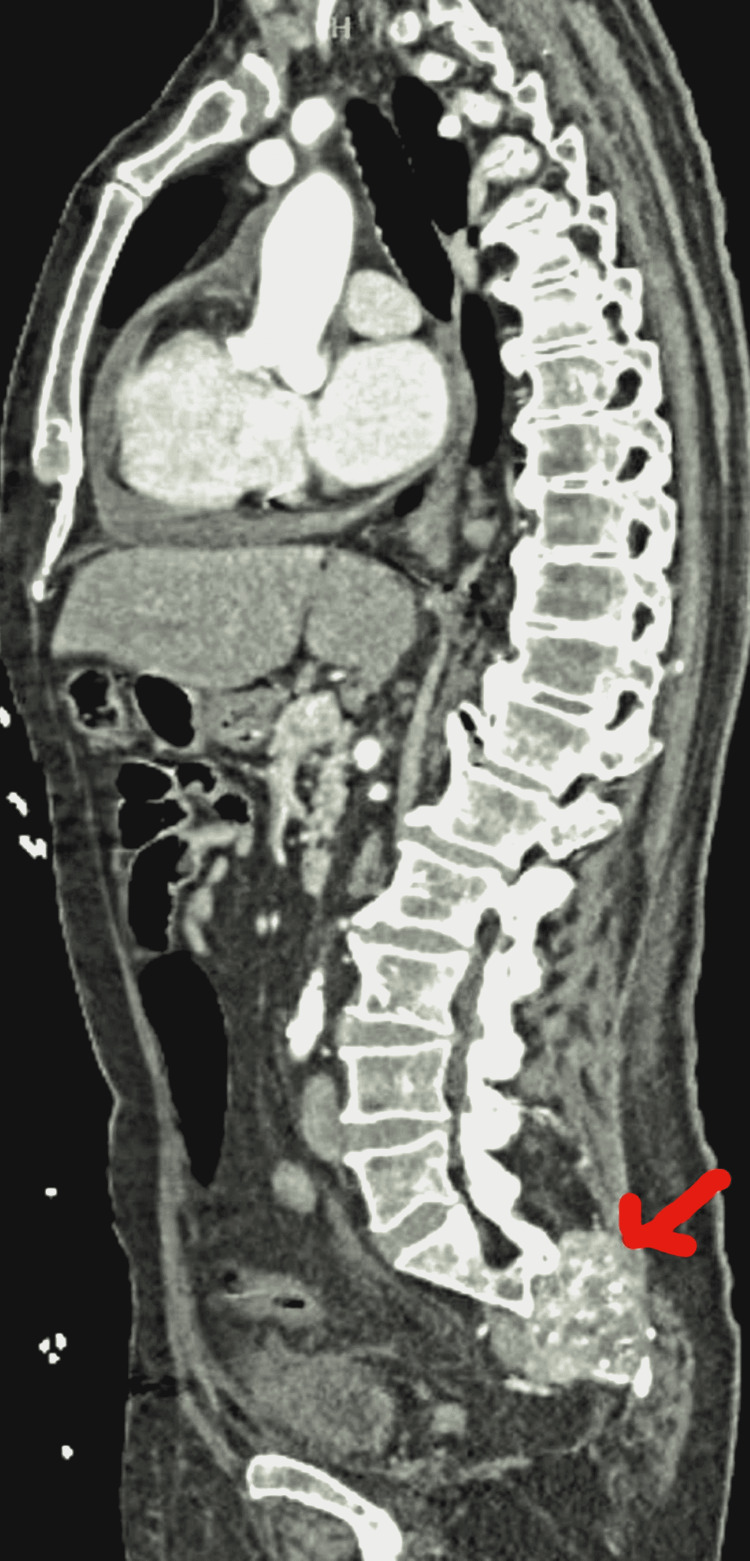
CT of chest, abdomen, and pelvis (sagittal view) revealing infiltrating sacral mass (red arrow)
